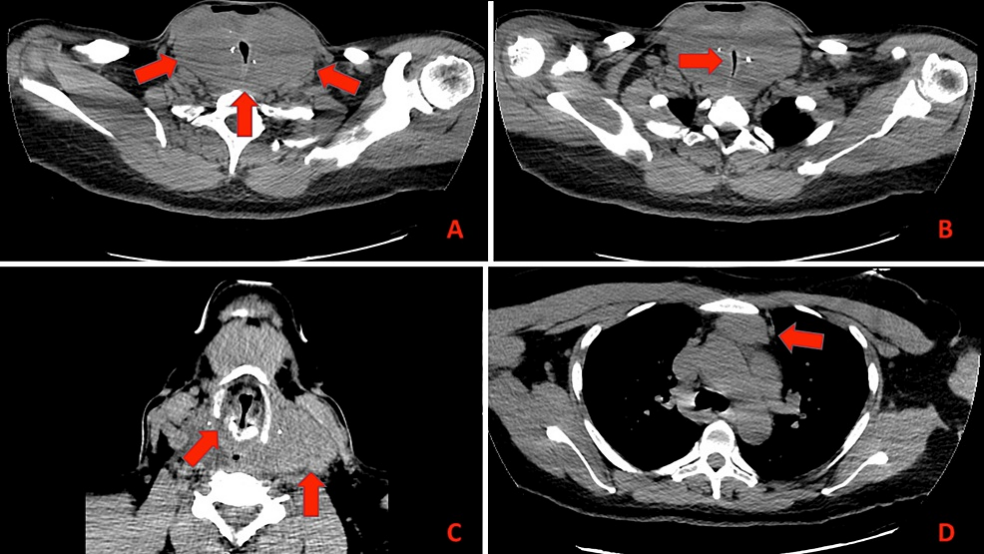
Computed tomography of the neck without contrast. (A) Large homogeneous lobulated soft tissue mass within the region of the thyroidectomy bed measuring approximately 6.5 x 12.2 x 6.2 cm. (B) Pronounced circumferential narrowing of the lower cervical and upper thoracic trachea. (C) Mass extends anteriorly and superiorly into the hypopharynx where there is ill-defined soft tissue within the right true vocal cord. (D) Mass extends inferiorly into the superior mediastinum where there is also a nodal agglomeration measuring 2.4 x 4.4 cm.
